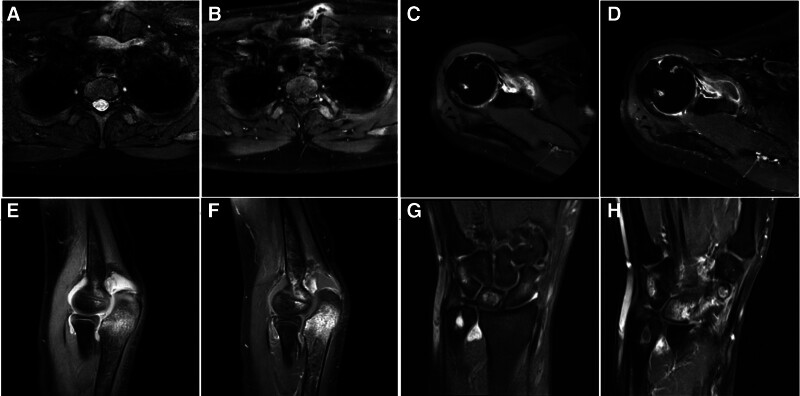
MRI image of brucellosis of the extraosseous joints of the spine. (A and B) MRI images of brucellosis in sternoclavicular joints. (A) The image of the transverse FS-T2WI plain scan. (B) The image of the transverse FS-T1WI enhanced scan. (C and D) MRI images of shoulder brucellosis. (C) The image of the transverse FS-T2WI plain scan. (D) The image of the transverse FS-T1WI enhanced scan. (E and F) MRI images of elbow brucellosis. (E) The coronal FS-T2WI plain scan image. (F) The coronal FS-T1WI enhanced scan image. (G and H) MRI image of brucellosis of the wrist joint. (G) The coronal FS-T2WI plain scan image. (H) The coronal FS-T1WI enhanced scan image. FS-T2WI = fat suppression-T2-weighted imaging, MRI = magnetic resonance imaging, T1WI = T1-weighted imaging, T2WI = T2-weighted imaging.
